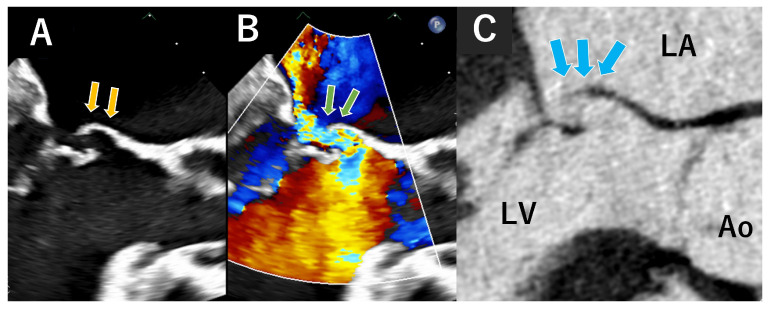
This is a case of infective endocarditis. Transesophageal echocardiography (TEE) revealed the anterior mitral leaflet aneurysm with perforation ((A): yellow arrows), which caused severe mitral regurgitation ((B): green arrows). Computed tomography showed an aneurysm and perforation of the mitral valve. Valve annulus abscesses were not suspected ((C): blue arrows). The mitral valve replacement was performed. LA; left atrium, LV; left ventricle, Ao; aorta.
